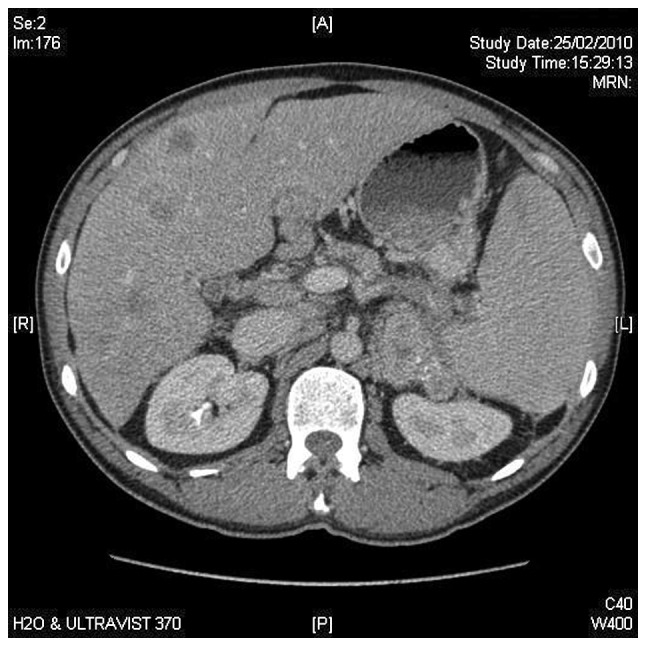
Abdominal CT images of patient 1, after two months of capecitabine/oxaliplatin therapy.
